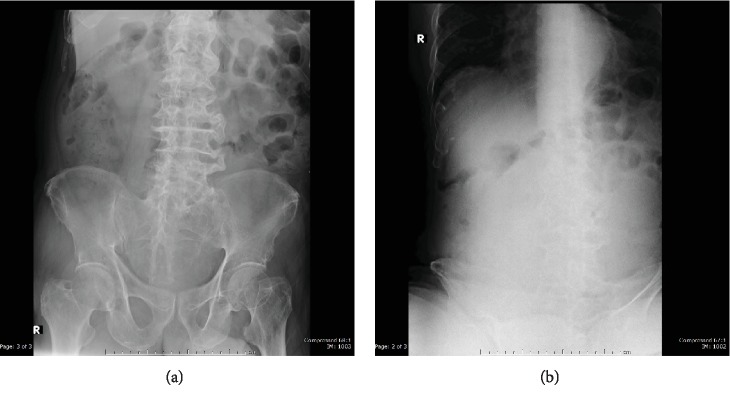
(a, b) Supine and upright abdominal radiographs show decreased amount of bowel gas in the lower abdomen/pelvic cavity.
